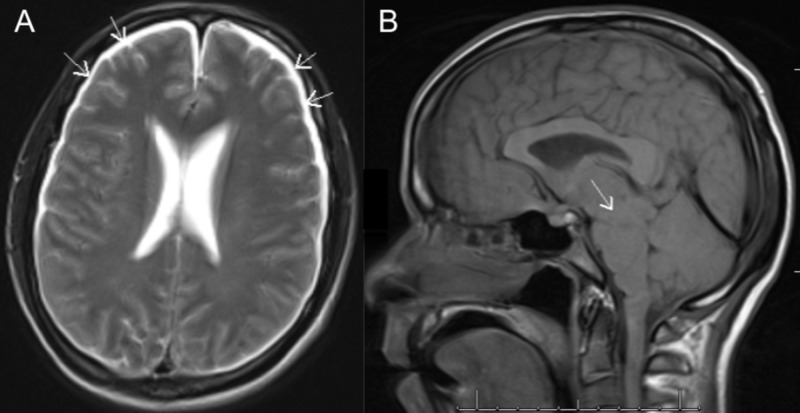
Initial brain magnetic resonance imaging (MRI) without contrast A) axial T2 images showing bilateral holohemispheric subdural effusions (arrows) with effacement of the sulci; B) sagittal T1 images, demonstrating distortion and downward displacement of the midbrain (arrow), most suggestive of cerebrospinal fluid (CSF) hypotension
